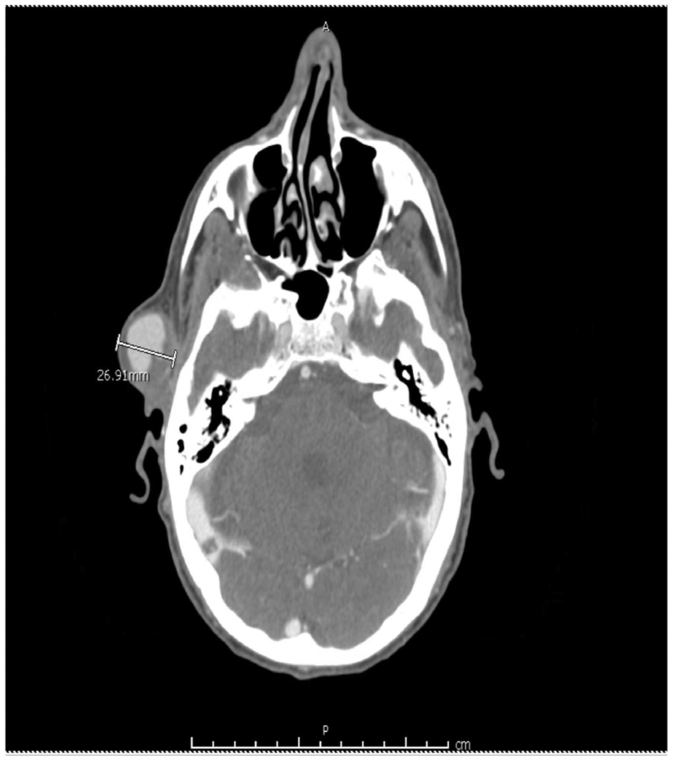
Computed tomography angiography demonstrates increased size of the right superficial temporal artery (STA) aneurysm to 2.69 cm.
